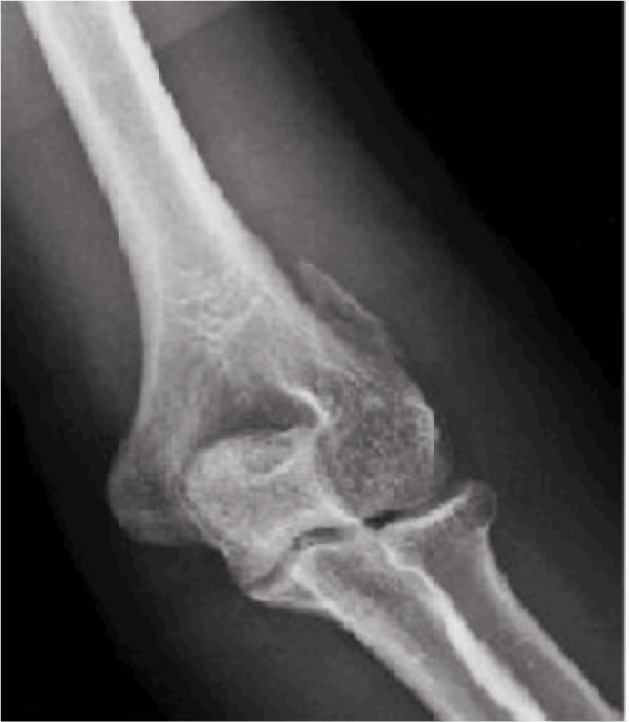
AP radiograph of left elbow avulsion fracture of brachioradialis origin.
